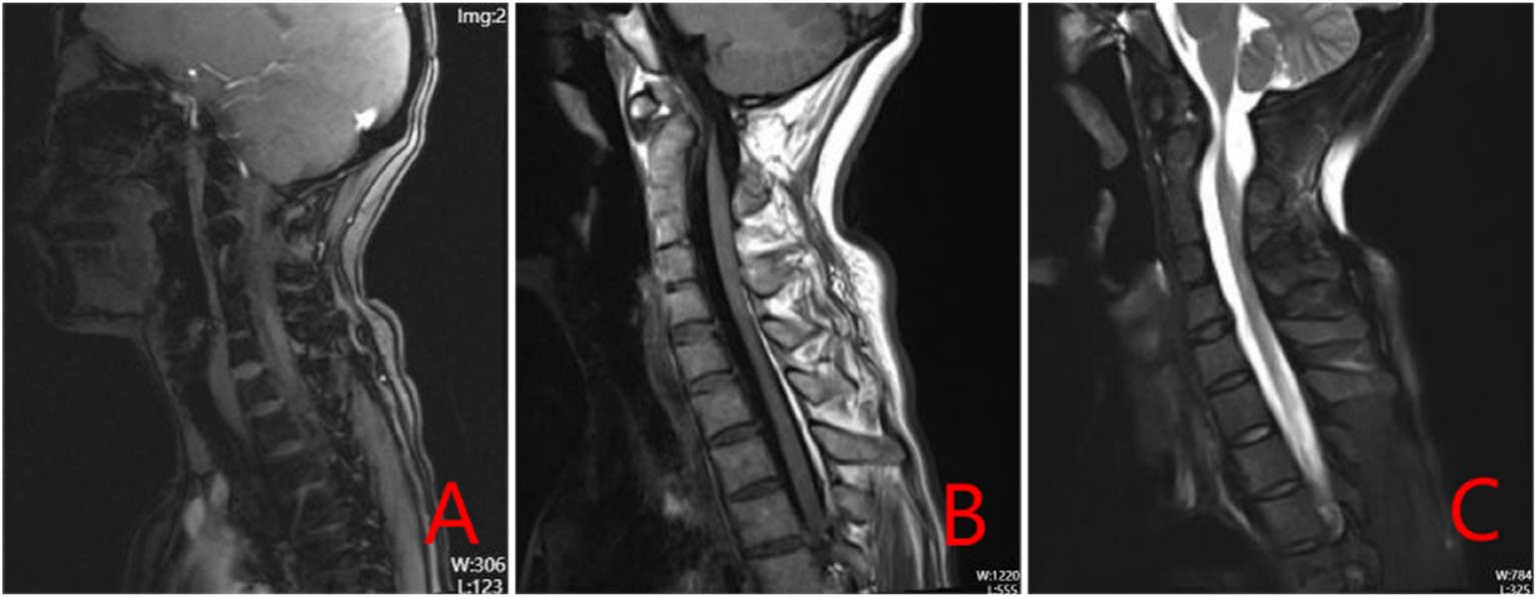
Patient's pre-operative sagittal MRI (A T1W1,B T2W1,C T2W1 STIR)
